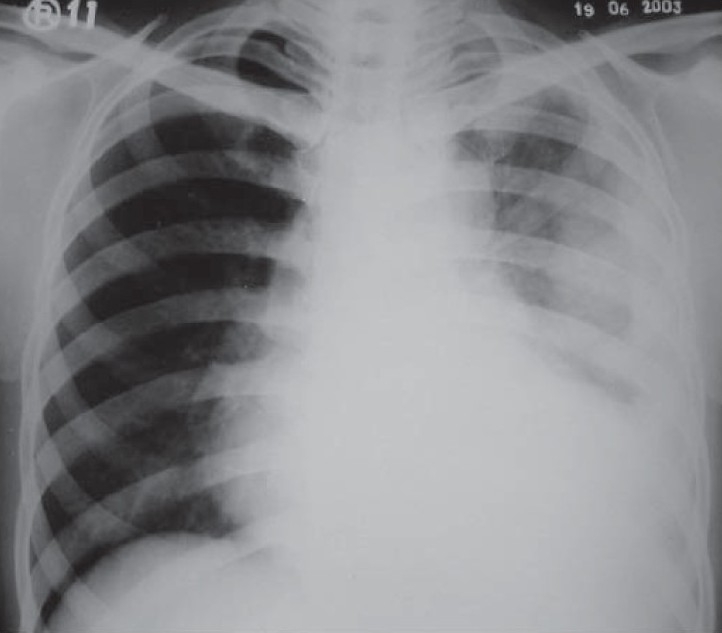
Chest radiograph shows inhomogeneous opacification of left hemithorax suggestive of left sided pleural thickening.
